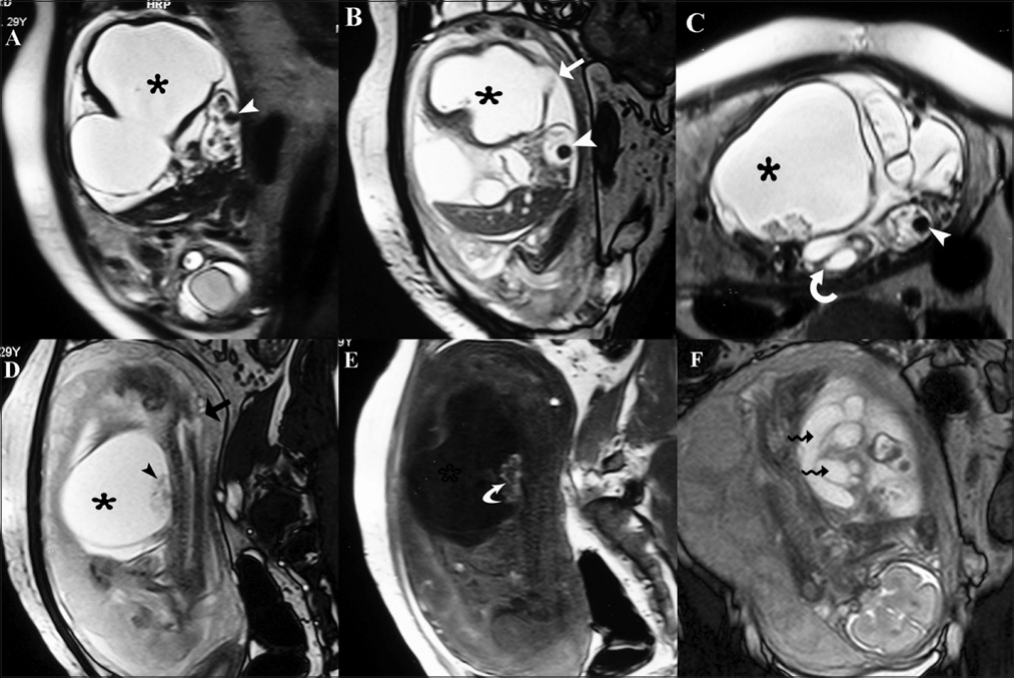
Antenatal MRI. Sagittal HASTE (A), Tru-FISP (B), and axial HASTE (C) images show a bilobed, cystic, abdominopelvic mass (asterisk) communicating with the dilated bowel (white arrow). Also, note the presence of enterolithiasis in the dilated bowel loop (white arrowhead in A-C). The bladder is not seen separate from the cystic mass. The right kidney is hydronephrotic (curved arrow in C). Sagittal Tru-FISP (D) and T1W (E) images show that the cystic lesion (asterisk) shows T2 hyperintensity and T1 hypointensity. Subtle T1 hyperintense foci suggestive of meconium are seen within the cystic mass (curved arrow in E). Also, note the presence of a lumbar meningocele (black arrow in D). In addition, debris is seen involving the cystic structure (black arrowhead in D). A sagittal Tru-FISP (F) image demonstrates the presence of a double ureter (wavy arrow) till the lower end; the ureters drain into the cystic lesion
